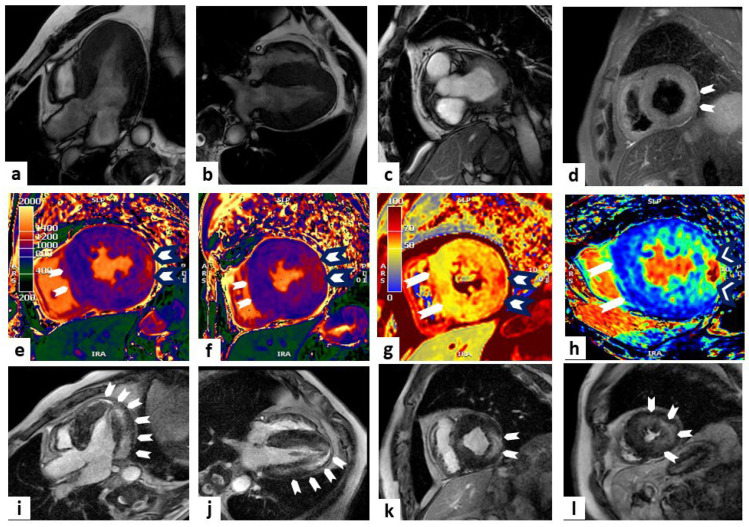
Anderson–Fabry disease. One case involves a 67-year-old man who was diagnosed with Anderson–Fabry disease through genetic testing during family screening (because of his affected brother). He remains completely asymptomatic. Cardiac magnetic resonance (CMR) imaging showed concentric LV hypertrophy with a mild reduction in ejection fraction (EF) in steady-state free precession (SSFP) sequences (panels (a–c) show three-chamber, four-chamber, and short-axis views at the basal level, respectively). Panel (d) shows oedema (white arrowheads) at the level of the mid-LV short-axis view (T2-weighted sequence). Panels (e,f) display T1 mapping sequences with a low value in the septum (white arrowheads) and higher values in the lateral wall (white arrowheads with black outlines) at the level of LV papillary muscles and the mid-LV short-axis view, respectively. Panels (g,h) display T2 mapping and extracellular volume (ECV) sequences, respectively, with low values in the septum (white arrowheads) and higher values in the postero-lateral wall (white arrowheads with black outlines) at the level of the mid-LV short-axis view. Late gadolinium enhancement (LGE) sequences display a non-ischemic hyperenhancement pattern (white arrowheads) at the level of the lateral wall (panels (i–l) show three-chamber, four-chamber, mid-LV short-axis, and apical LV views, respectively).
